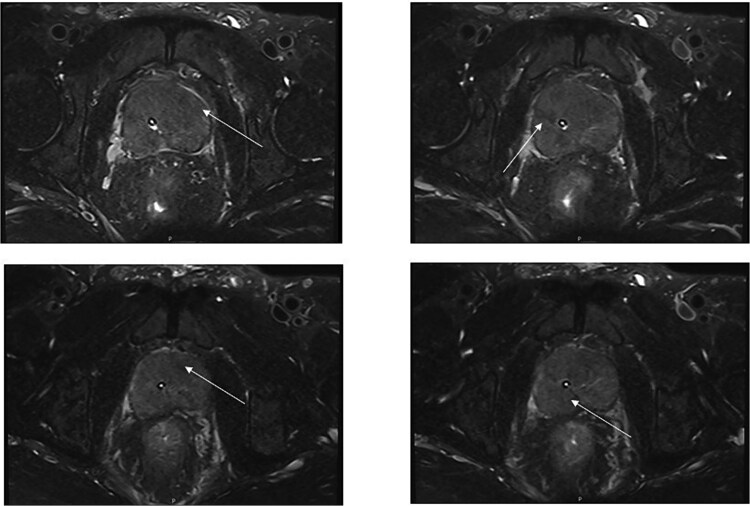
Prostate MRI: The prostate is approximately 53mm × 46mm × 54mm at most; the prostate volume is enlarged and the contour is irregular. The T2WI signals of the bilateral peripheral zones and transition zones of the prostate are significantly decreased and decomposed unclearly, accompanied by the formation of masses (arrows). The urethra deviates to the right; the prostate capsule is intact. The spermatic glands on both sides were not displayed clearly, and the T2WI signal was unevenly decreased. The PI-RADS score is 5. Prostate cancer (mrT3N1Mx) is considered.
